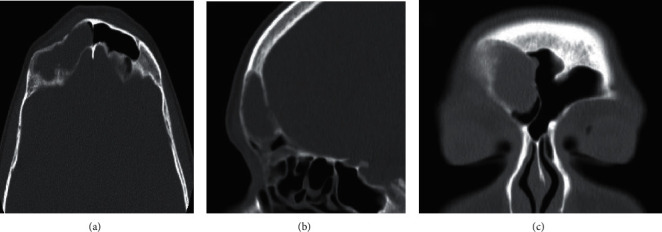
Computed tomography of paranasal sinuses in a bone algorithm in axial (a), sagittal (b), and coronal (c) planes. Right frontal sinus lesion with depressed fracture of the anterior wall and resorption of the lateral and posterior walls.
